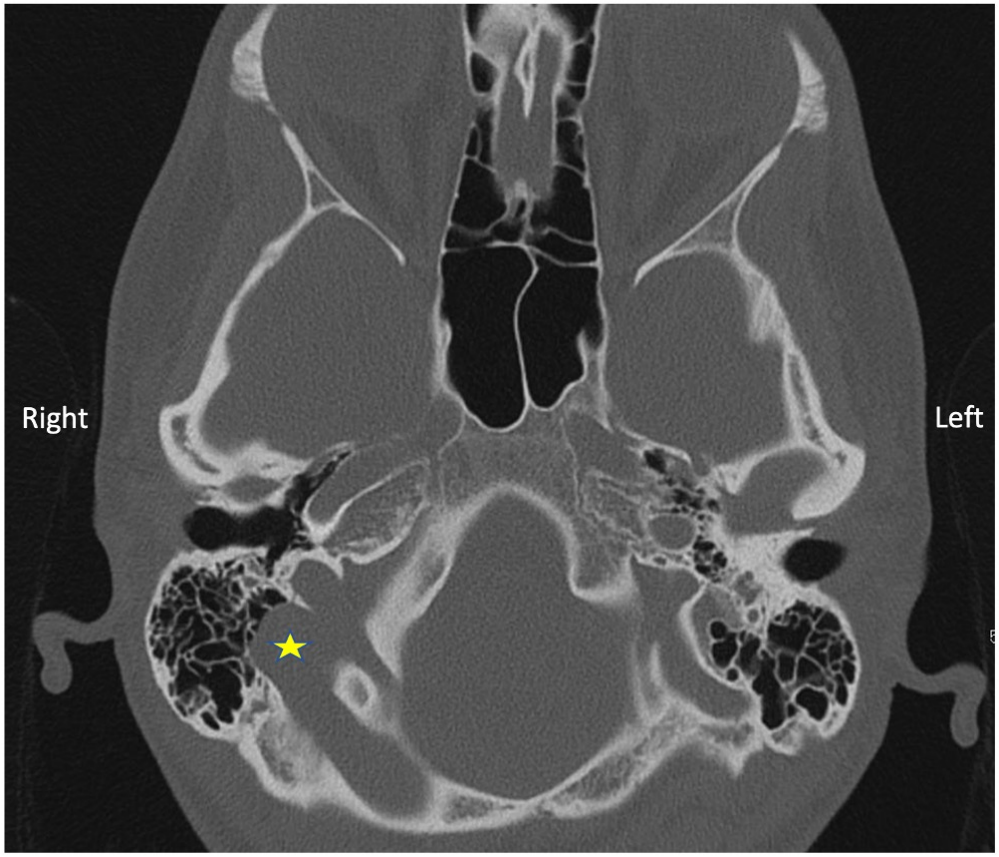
CT scan showing the right high jugular bulb, dehiscent sigmoid plate with sigmoid sinus herniation into the mastoid (yellow star)
